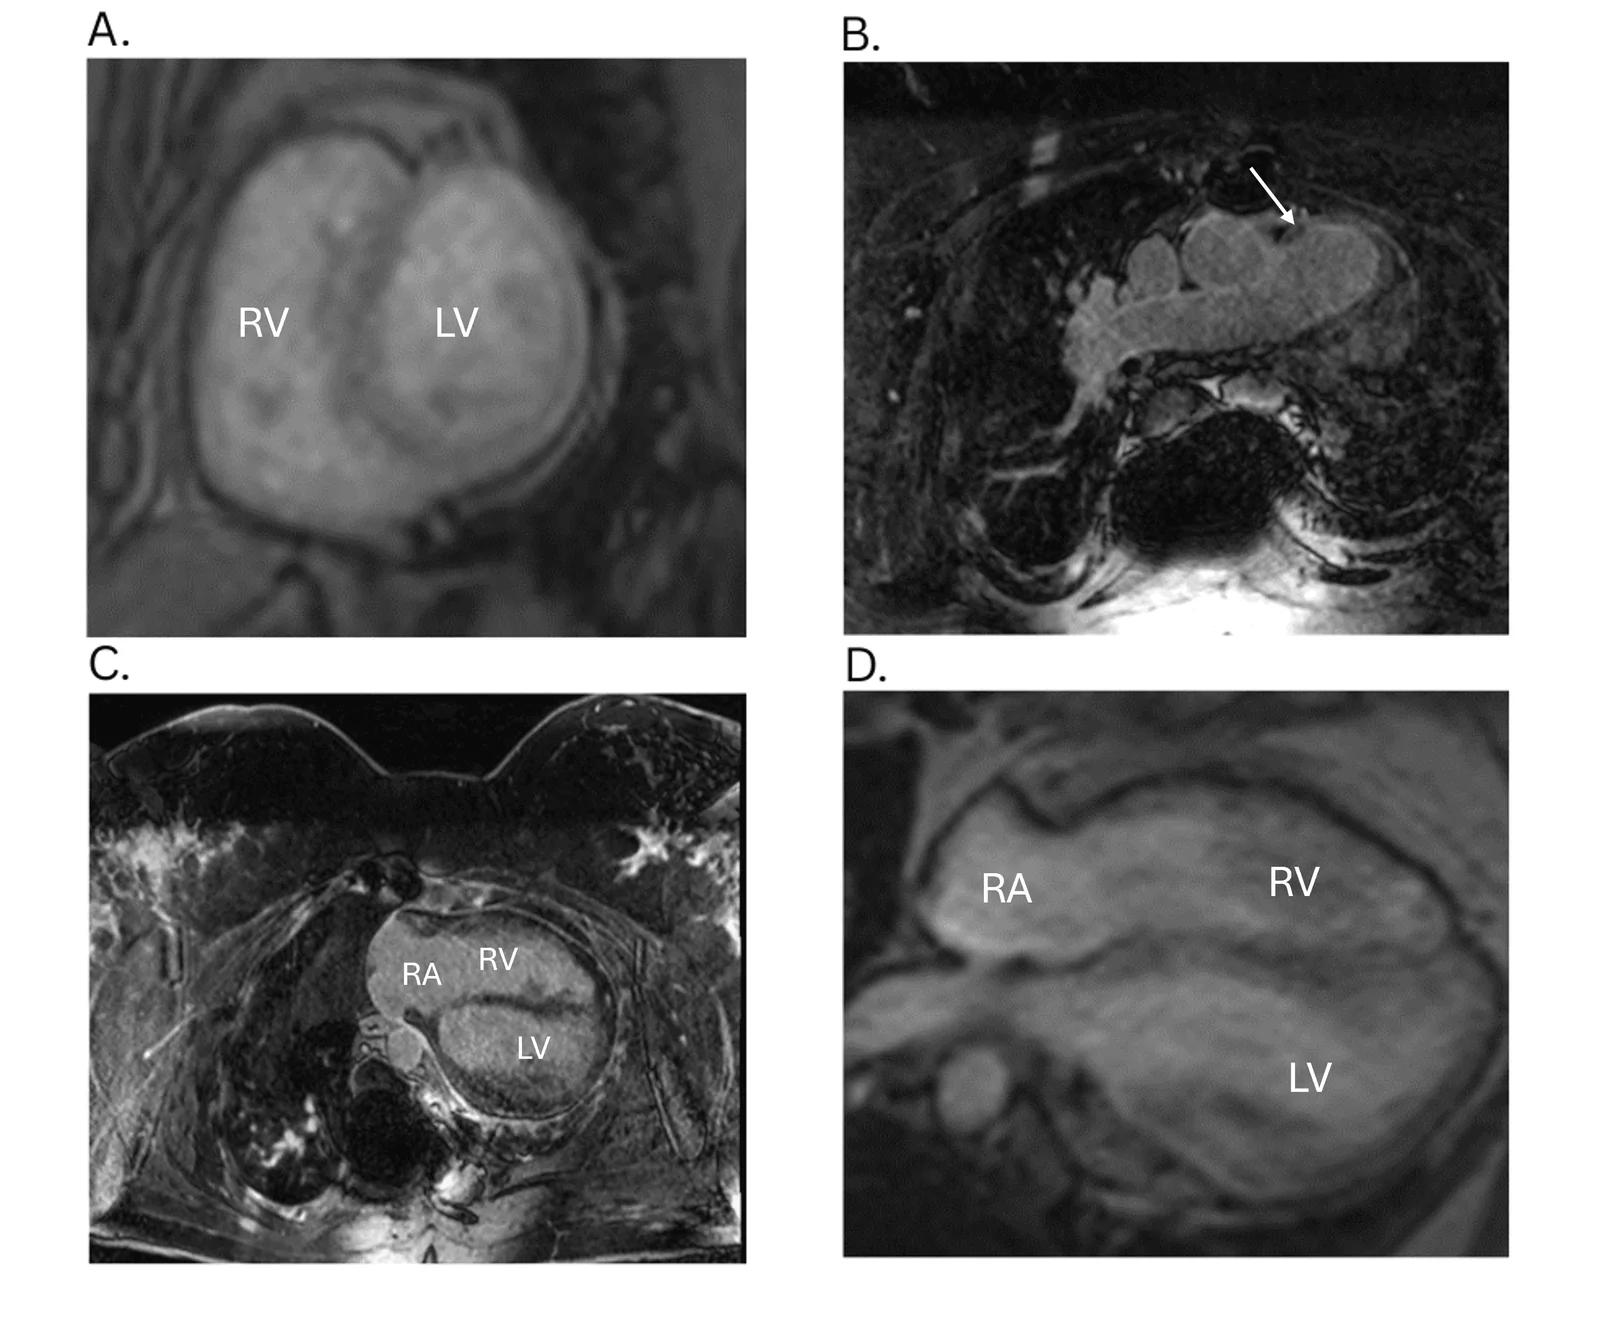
Cardiac MRI findings in Case 4. Cardiac magnetic resonance (CMR) images include a real-time spoiled gradient-echo short-axis image (A), 3D delayed postcontrast axial images (B and C), and a balanced steady-state free-precession four-chamber long-axis image (D). The images demonstrate main pulmonary artery and biventricular enlargement, mildly decreased right ventricular systolic function (ejection fraction = 40%), and normal left ventricular systolic function (ejection fraction = 55%). No delayed myocardial enhancement is present. RA: Right atrium; RV: Right ventricle; LV: Left ventricle; Arrow points to the main pulmonary artery.
